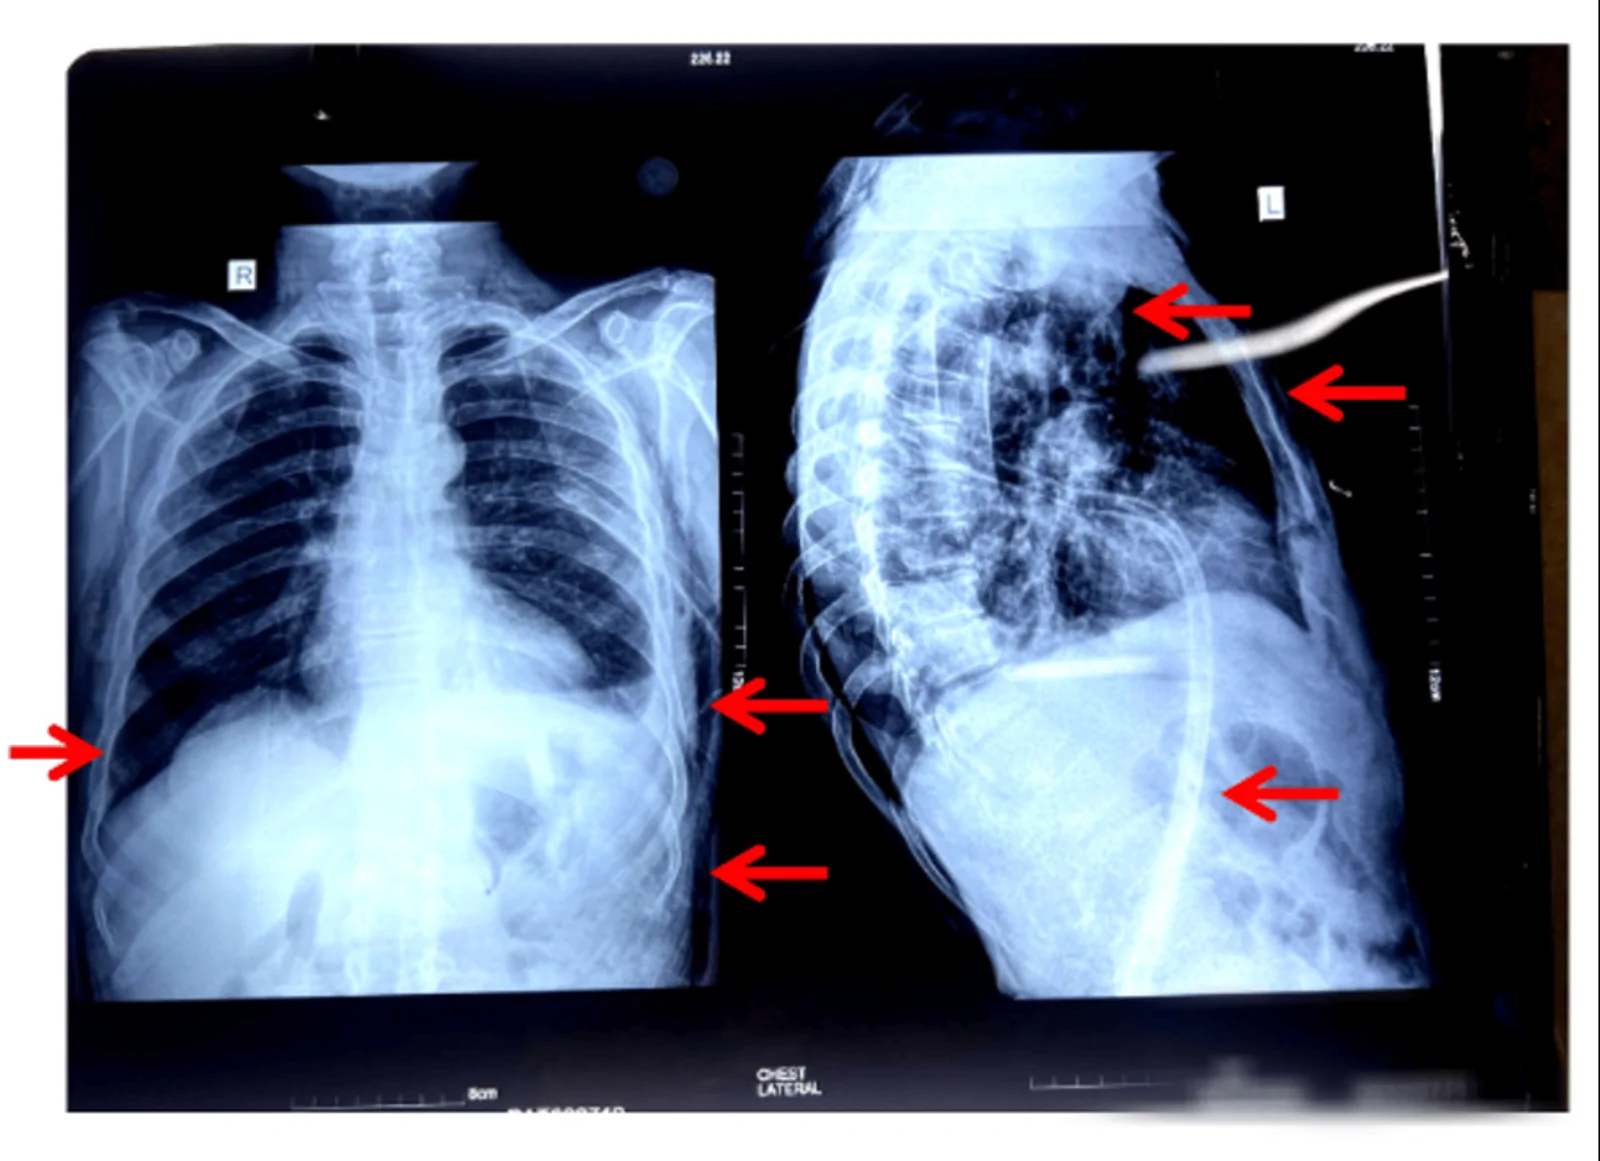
Chest X-ray showing pneumomediastinum with subcutaneous emphysema Posteroanterior chest radiograph demonstrating extensive pneumomediastinum with arrows representing subcutaneous emphysema involving the cervical and thoracic soft tissues. Additionally, no evidence of pneumothorax is identified.
